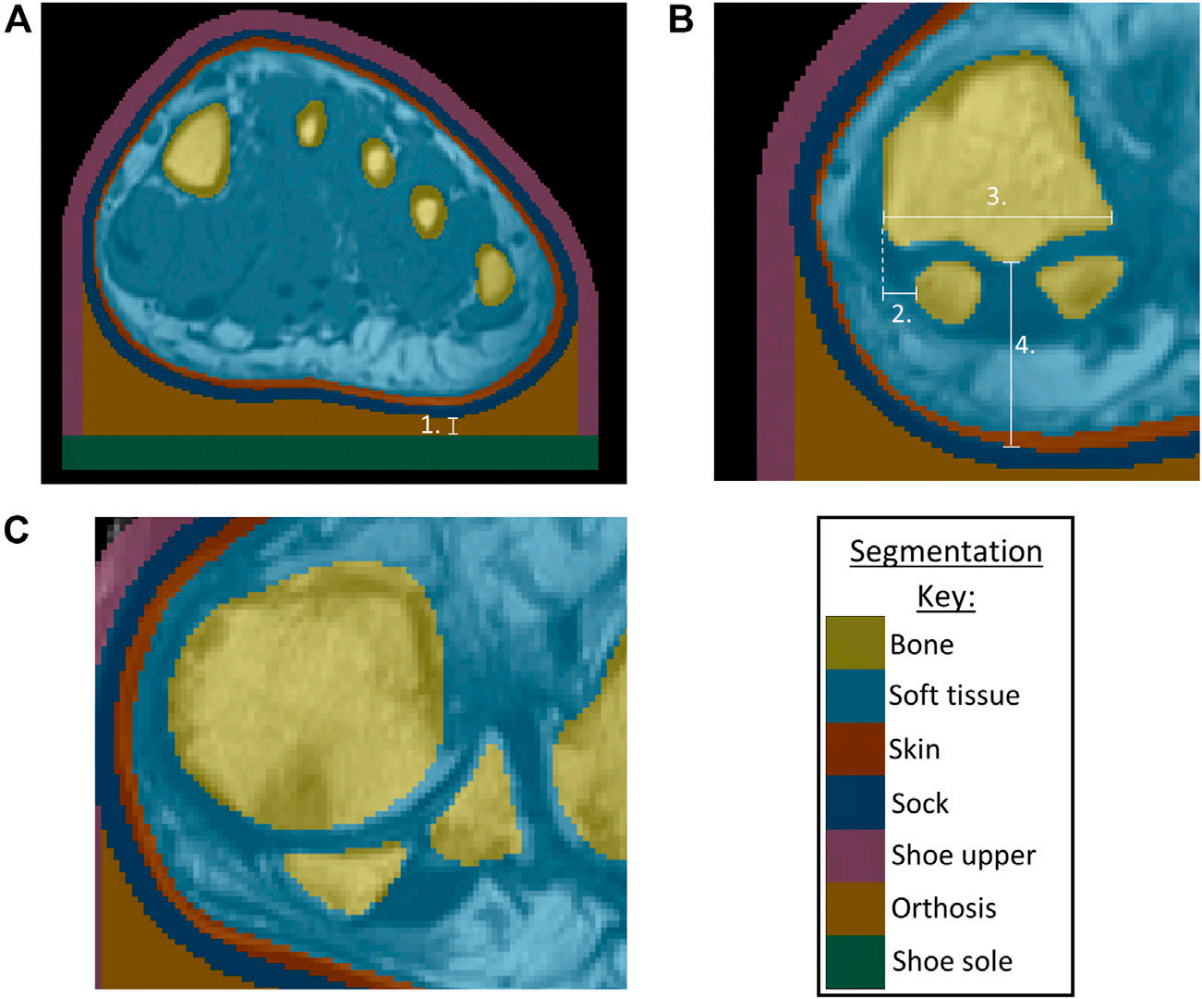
(A) Segmentation of bones, skin, soft tissue from P3 MRI, with addition of sock, shoe upper, orthosis, shoe sole, indicating minimum orthosis depth (1.) (B) Morphological measurements for P3 showing sesamoids in a normal position, with 2. Lateral distance from MH1 edge to sesamoid, 3. MH1 width, 4. Tissue depth under MH1 (C) P1 MRI showing example of sesamoids in a displaced position.
